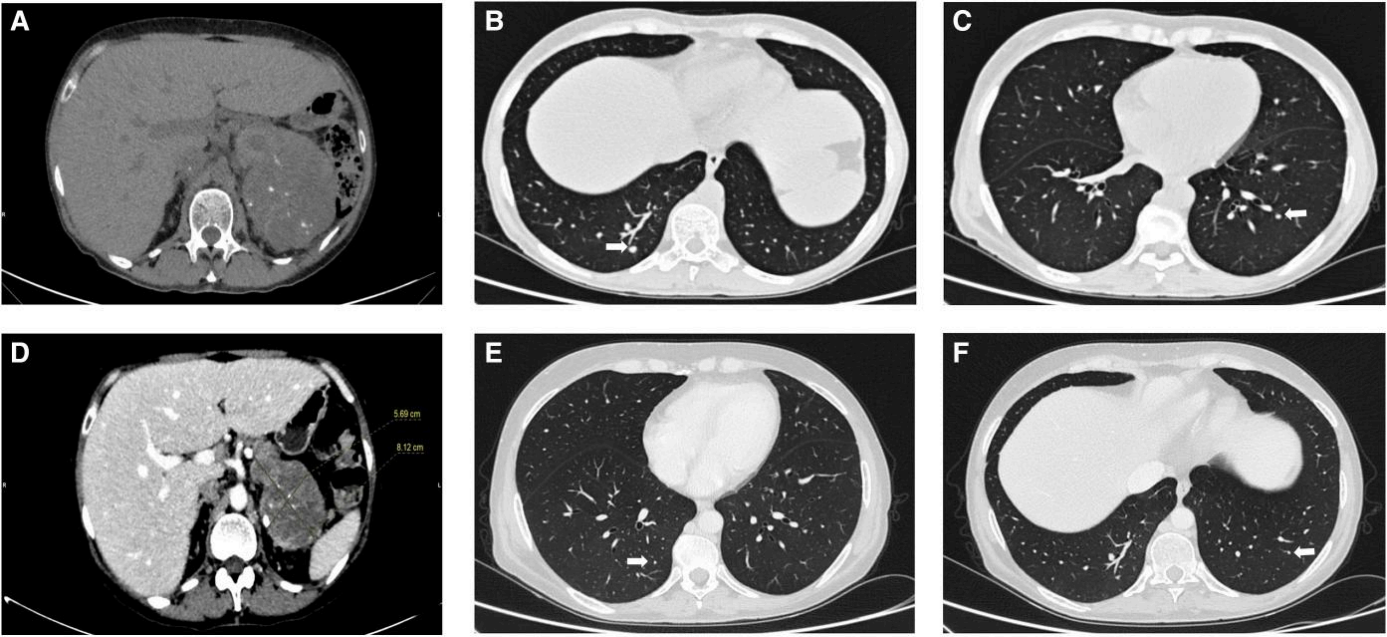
A contrast-enhanced computed tomography scan documented a reduction of the primary tumor (maximum diameter of 11 cm) (A) and in size of lung bilateral metastatic foci 4 months after starting mitotane (B, C). Follow-up imaging performed after 8 months after mitotane treatment showed an impressive regression of adrenocortical carcinoma (maximum diameter 8 cm) (D) and the lung metastatic nodules (white arrows) (E, F).
